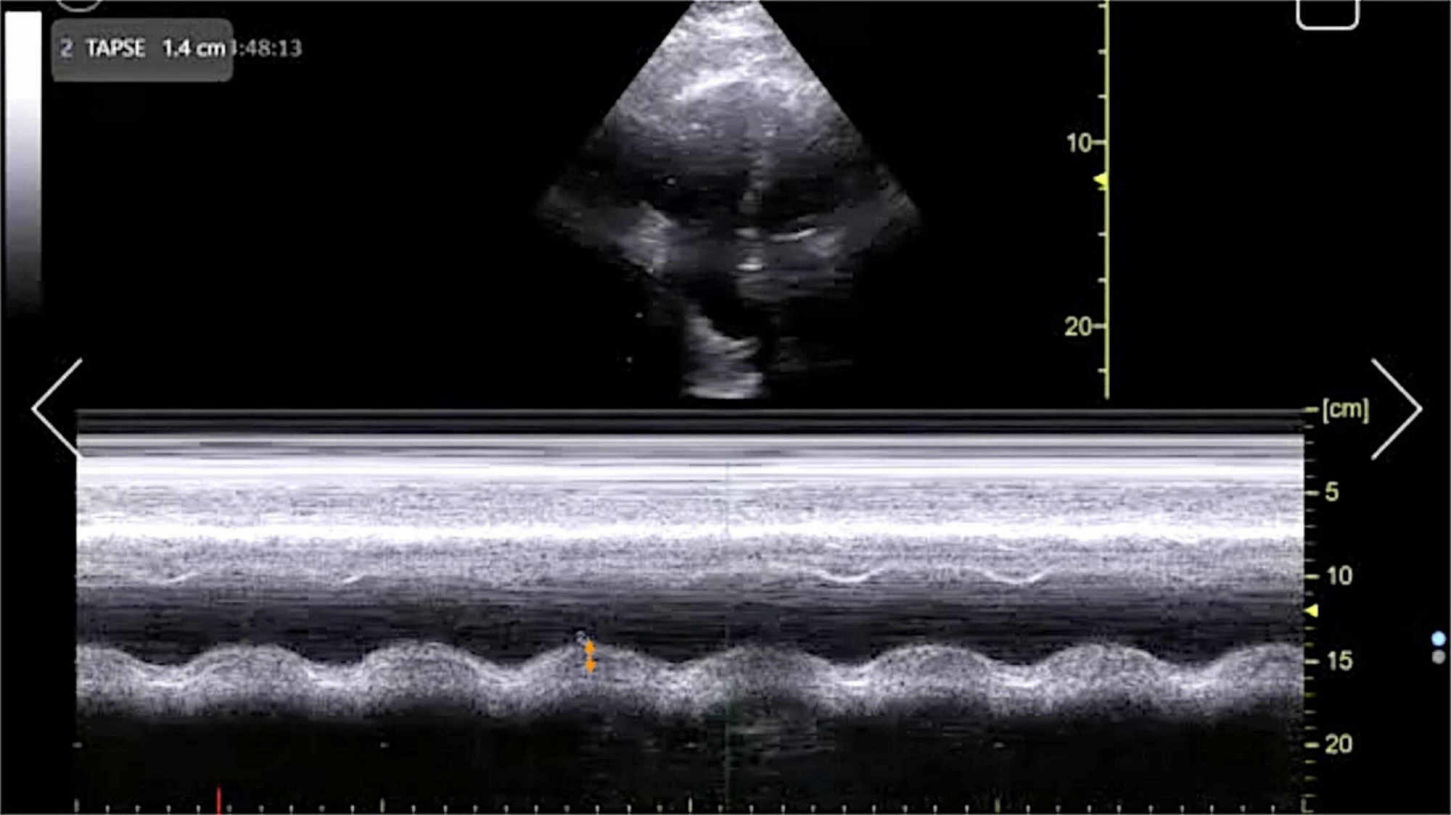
Point-of-care echocardiography two hours post-thrombolysis. Apical four-chamber view with M-mode tracing demonstrating TAPSE (orange arrow). TAPSE, tricuspid annular plane systolic excursion.
